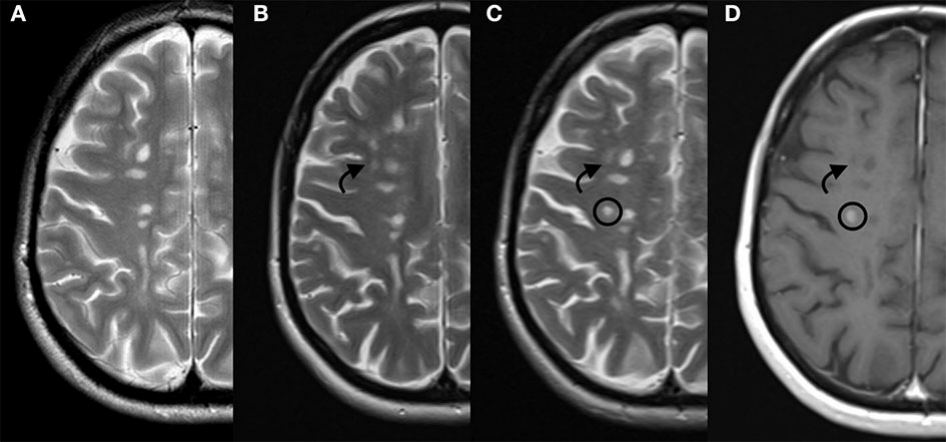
Patient's disease progression on MRI. From the left to the right: T2 weighted sequences respectively on June 2018 (A), February 2019 (B), and July 2019 (C), and; contrast enhanced T1 weighted sequence on July 2019 (D). Arrow indicates a new demyelinating lesion and circle indicates a new demyelinating lesion presenting contrast enhancement. MRI, magnetic resonance imaging.
